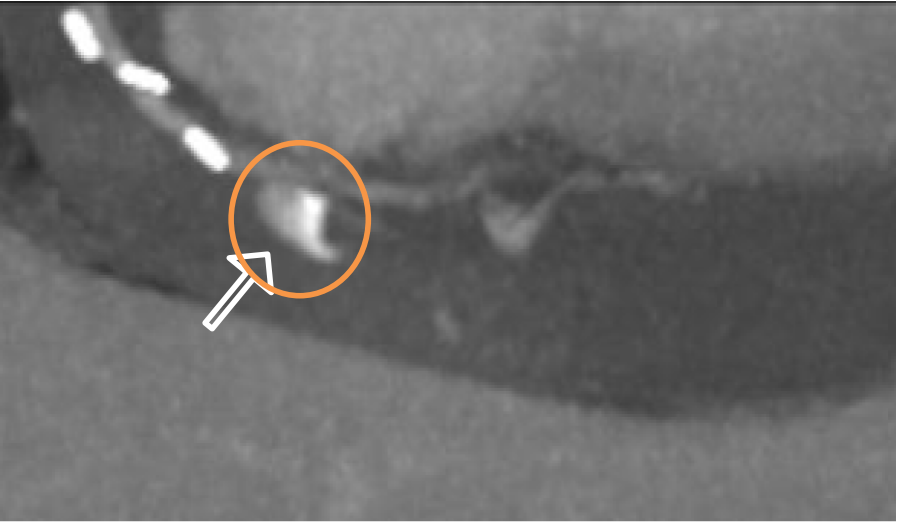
Active extravasation of contrast agent on abdominal CT Scan with blood pooling in portalvenous phase, paracoronal plane with MIP- reconstruction. (CT: computer tomography; MIP: maximum intensity projection)
